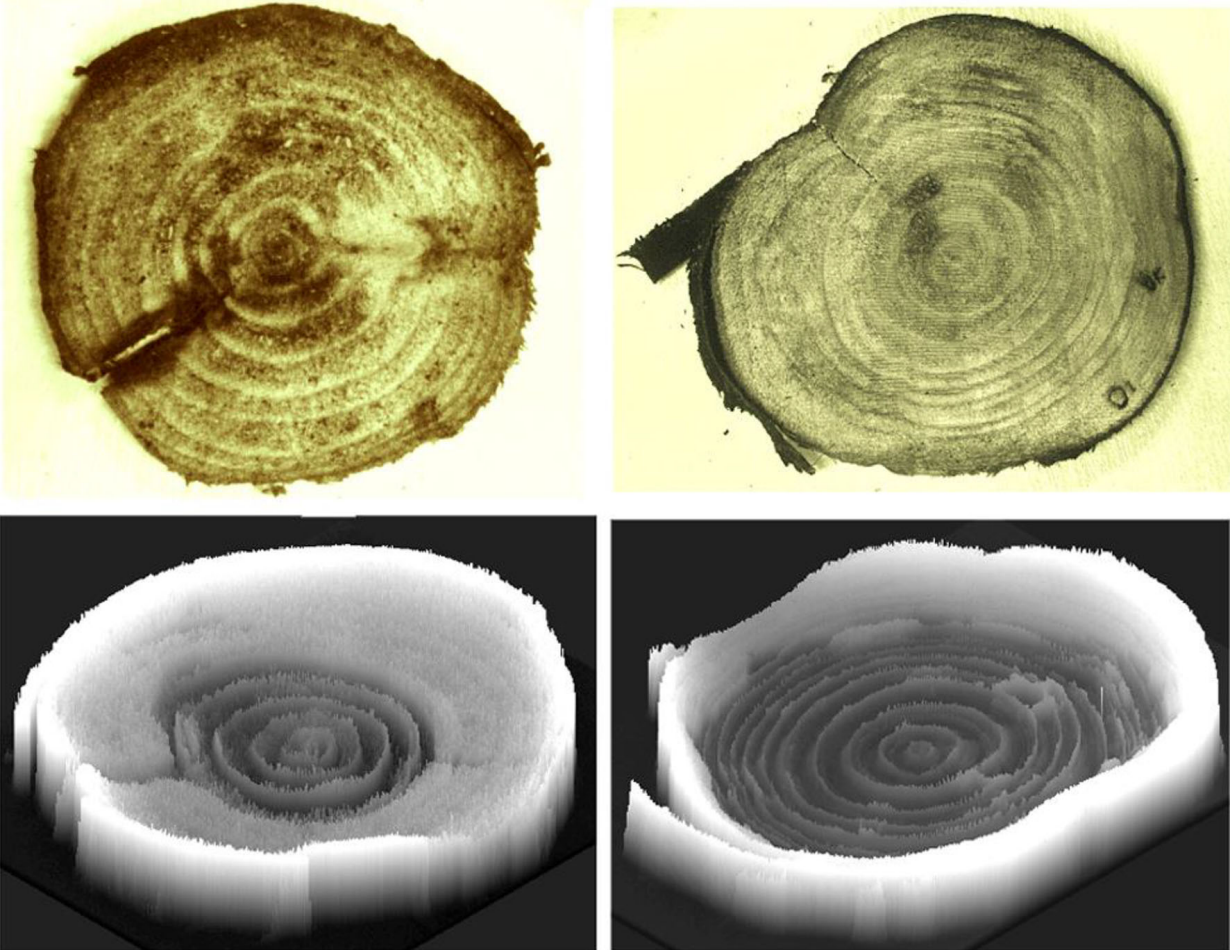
Water distribution of acacia and Japanese cypress. *Note* The upper two images are pictures and the lower two images are neutron beam images. *Left* acacia, *right* Japanese cypress. Wood discs, 1 cm in width, were prepared just before neutron beam irradiation. The whiteness of the image obtained in an X-ray film after irradiation corresponded to the water image; therefore, the 2-dimensional image was converted to a spatial one where the height was the degree of the whiteness representing the water content. In the case of acacia, heartwood formation could not be confirmed form the picture because it did not change any color. However, the lower picture showed clear difference of the water distribution between heartwood and sapwood parts. Water image of the Japanese cypress showed high amount of water in only outer part of the wood and small amount of water was distributed along the annual ring in inner part
